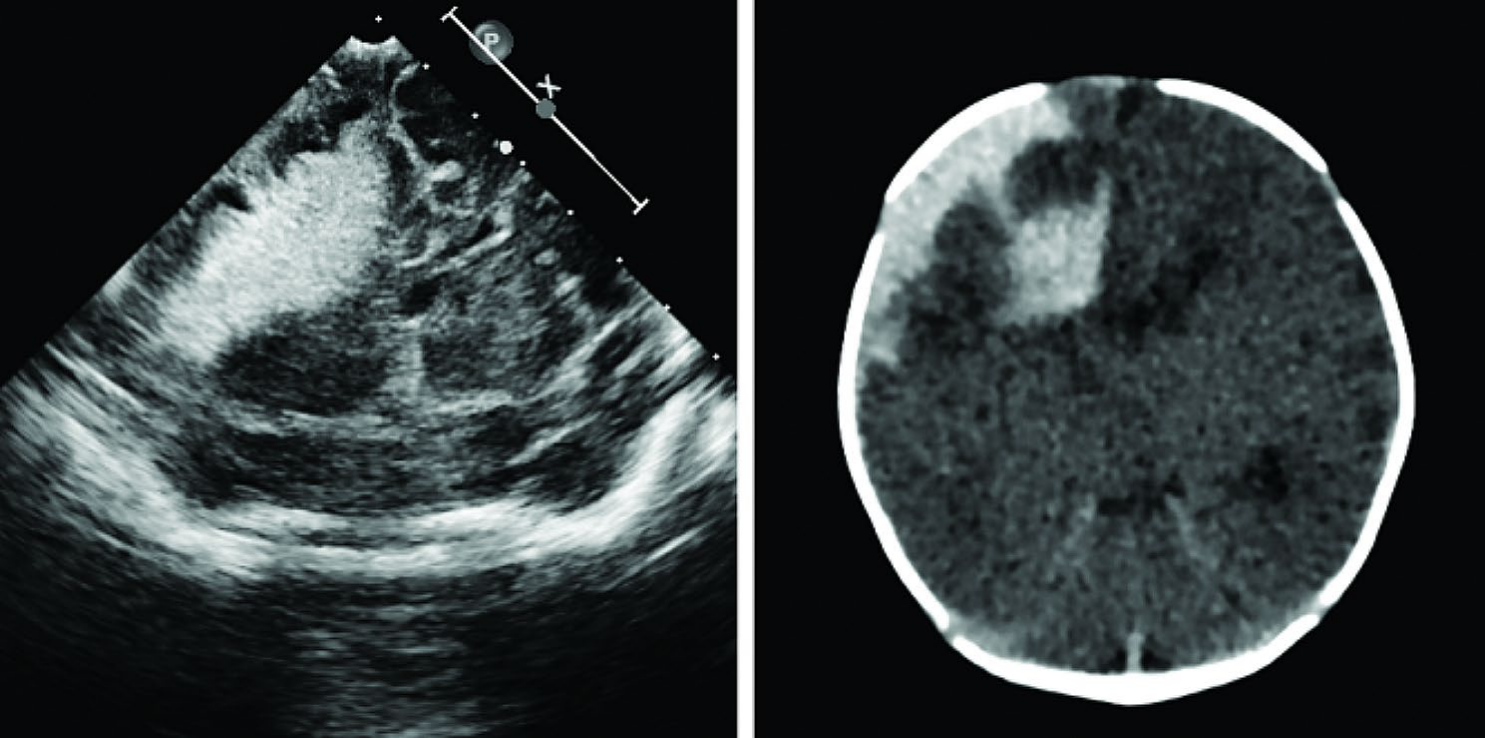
Diagnosis of intracranial hemorrhage. Left: Coronal transfontanelle ultrasonographic image showing large hyperechoic mass in the right cerebral hemisphere causing midline shift. Right: Coronal noncontrast CT image showing right intraparenchymal hemorrhage with surrounding edema and midline shift. There is also right subarachnoid hemorrhage
